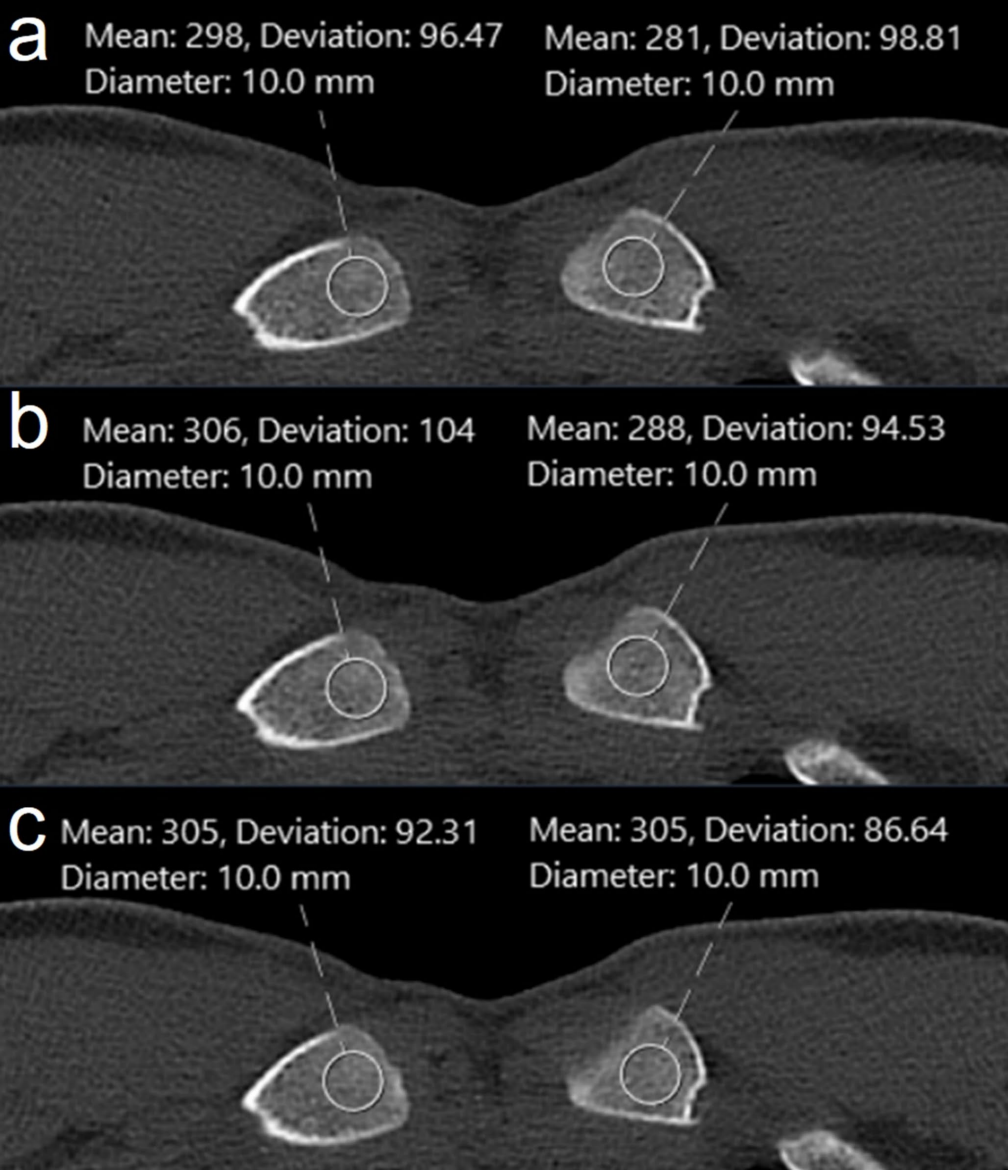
Noise measurements for bone. To measure CT image noise in bone, single 10 mm ROIs were placed within the MCE on three consecutive images of the right and left air clavicle. Great care was taken to exclude cortical bone from the ROIs
